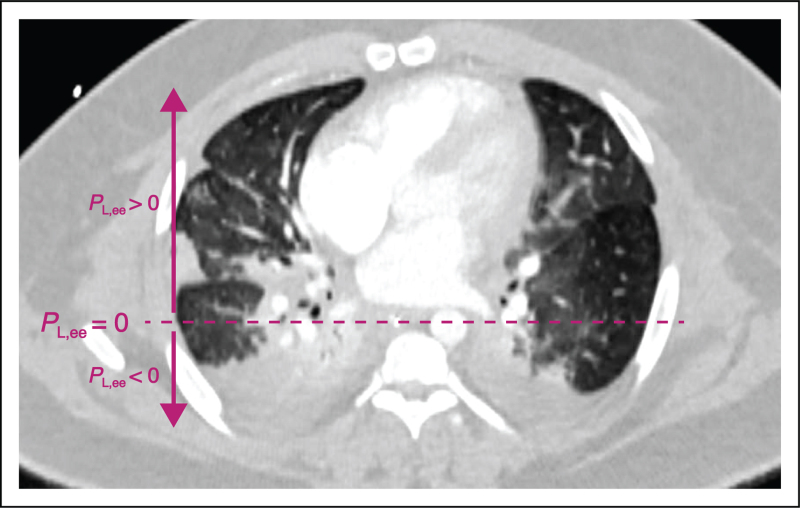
CT scan of a patient with acute respiratory distress syndrome due to COVID-19. The spatial gradient of *P*_L_ is aggravated with heterogeneous lung injury. Note that a targeted *P*_L,ee_ of 0 cmH_2_O in supine position will reflect the *P*_L_ at the level of the esophagus; the *P*_L_ will be negative in the areas dorsal to the esophagus, and positive in the ventral parts of the lungs.
